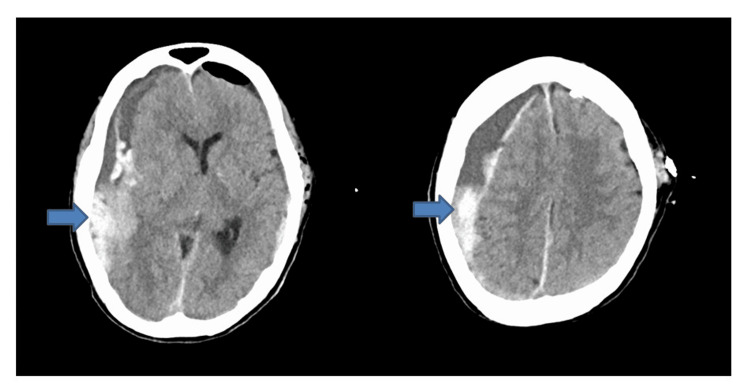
Follow-up CT performed nine hours and 27 minutes after arrival showed further enlargement of the right hematoma (30 mm thick) (arrow) with a 13 mm midline shift to the left, while the left side remained clear.
